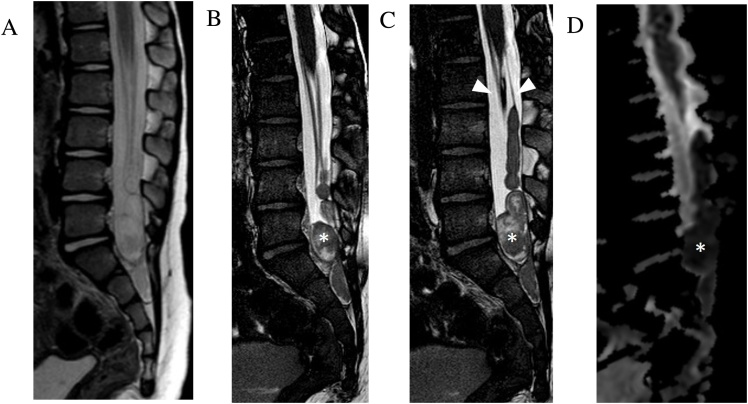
3-month-old male with congenital spinal dysraphism. Sagittal T2-weighted FSE (a), FIESTA-C (b–c) and ADC (d) demonstrate thickened tethered bands (arrowheads) and multiple masses (asterisks) in the caudal thecal sac which are better seen on FIESTA-C images. ADC image demonstrates reduced values within the masses compatible with epidermoid cysts.
